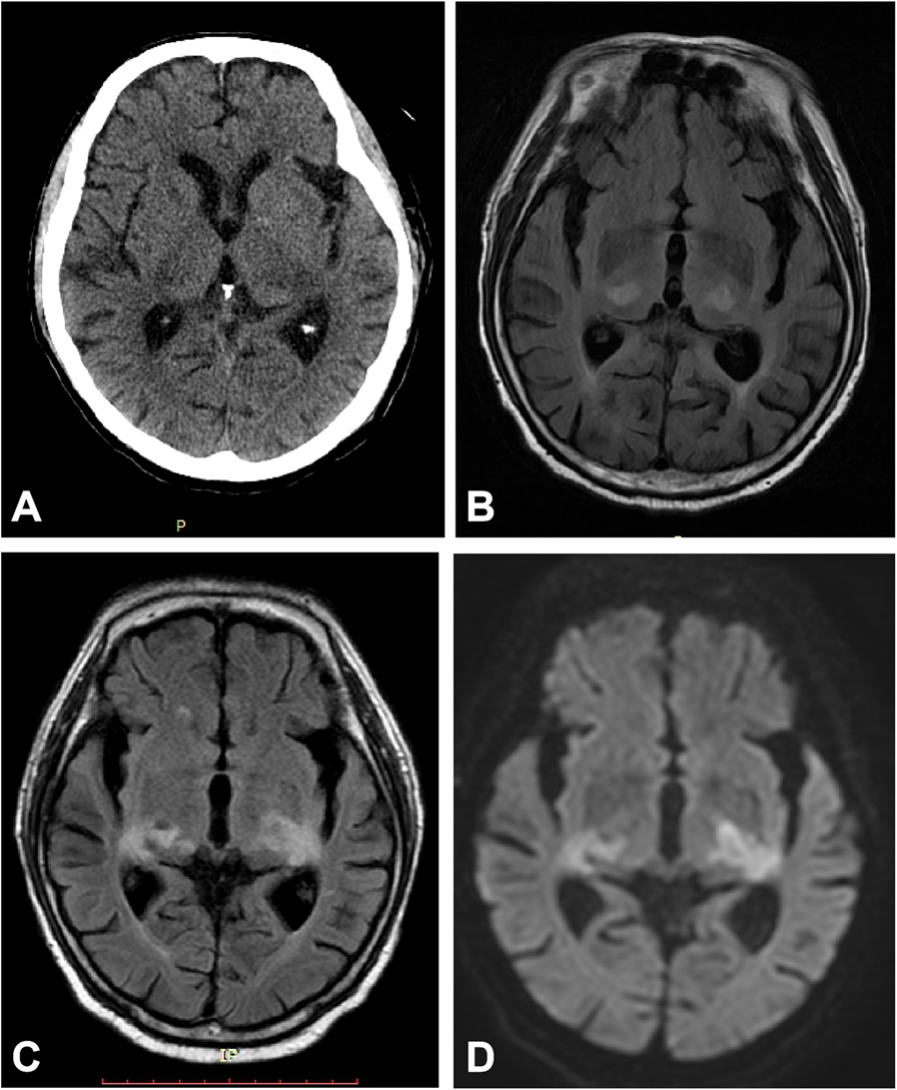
CT and MRI scans showing bilateral thalamic lesions. The CT scan of the head shows low density changes in the bilateral thalamic lesions (a). Axial FLAIR image reveals symmetric hyperintense signal alterations in thalami (b) and enlargement of the lesions after 17 days (c) with mild hyperintensity changes in DWI (d)
